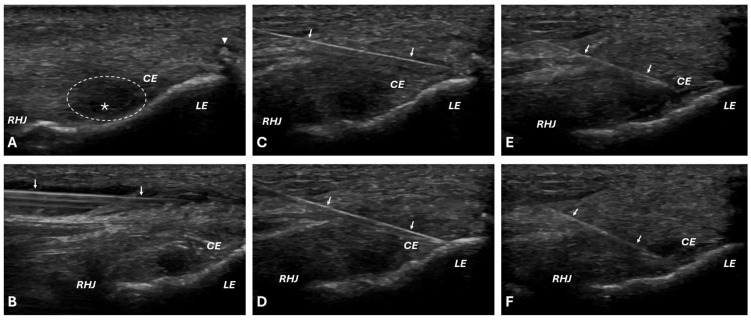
Presentation of the ultrasound-guided injection technique: (A) ultrasonographic image of pathological changes in lateral epicondyle tendinopathy (tendon edema, hypoechoic area, partial tear, bony spurs); (B) needle positioning during the administration of anesthetic; (C–F) injection using a multiple-needle puncture technique from proximal to distal, extending from the deep forearm fascia to the periosteum (D), with medication administered during needle withdrawal (E) and directly into the visibly damaged area (F). Arrow, needle position; arrowhead, bony spurs; asterisk, tendon tears; CE, common extensor origin; dotted-line circle, hypoechoic area; LE, lateral epicondyle; RCJ, radiohumeral joint.
